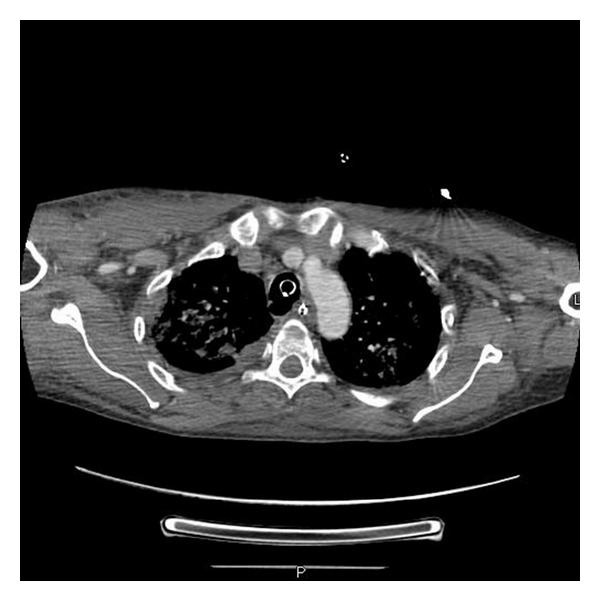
CT scan of chest mediastinal view showing endotracheal tube in trachea and also showing weakness in the posterior membranous wall of trachea.
